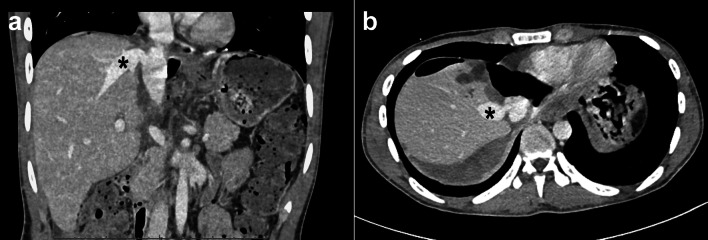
Postoperative enhanced computed tomography showing the right hepatic vein graft (asterisk) and no stenosis in the inferior vena cava (a coronal section, b axial section)
